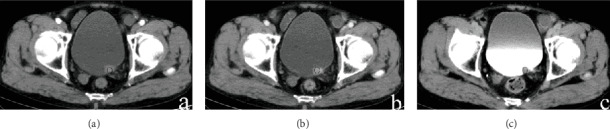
(a–c) A 45-year-old man had bladder cancer that presented as nodule-like lesions in the left trigone. The enhanced CT values of the lesions in the arterial, venous, and delayed phases were 76.2, 81.5, and 76.4 HU, respectively. The enhanced CT values of the bladder wall in these three phases were 32.1, 34.3, and 31.6 HU. The relative enhanced CT values (calculated as enhancement CT values of the lesion − enhancement CT values of normal bladder) in the arterial, venous, and delayed phases were 41.2, 41.5, and 46.4 HU, respectively.
